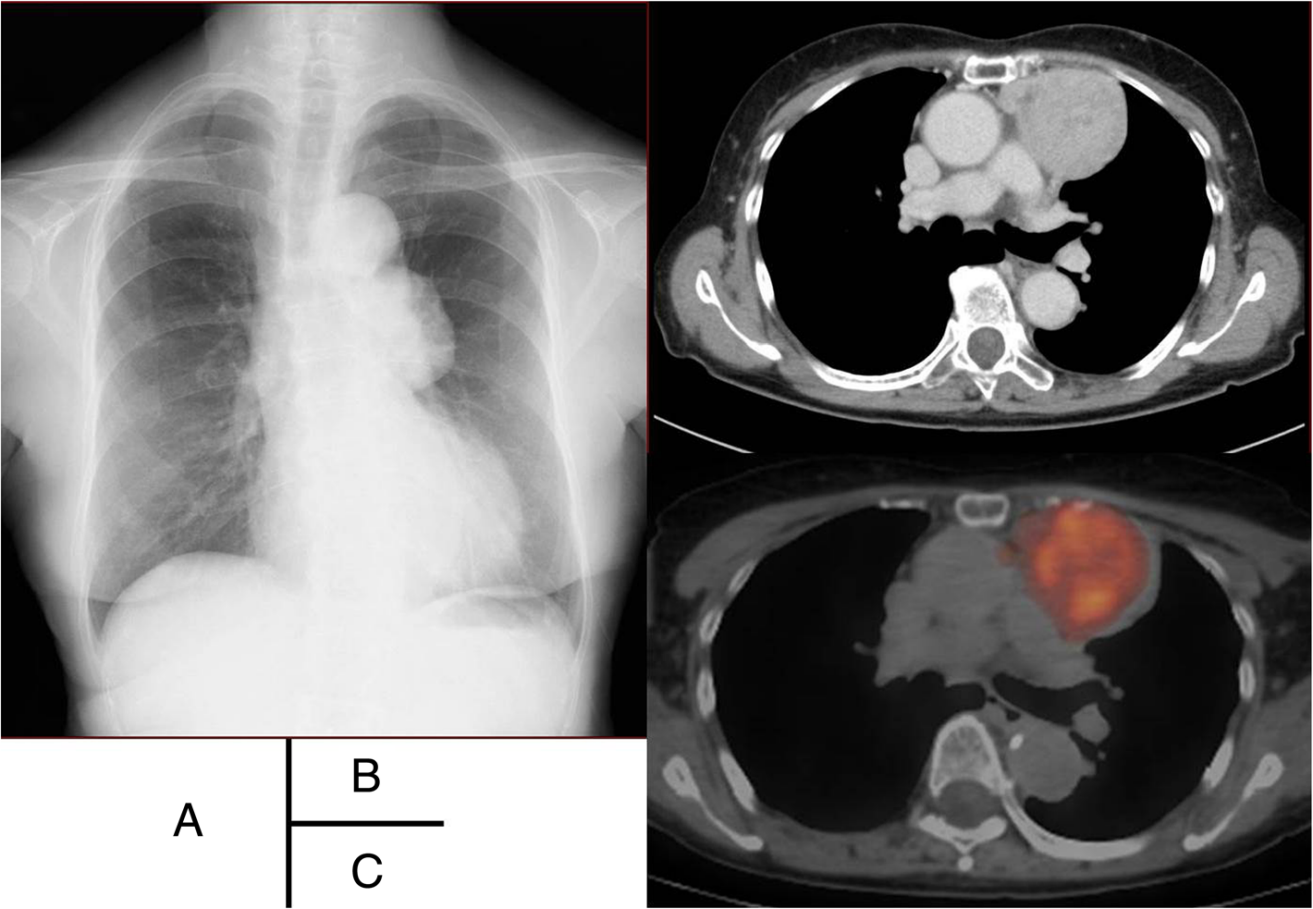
Chest X-ray showing an abnormal shadow in the mediastinum (a). Contrast-enhanced chest computed tomography showing a large round mass not invading the surrounding organs in the anterior mediastinum (b). Fluorodeoxyglucose positron emission tomography showing a mild hypermetabolic mass in the anterior mediastinum (c)
